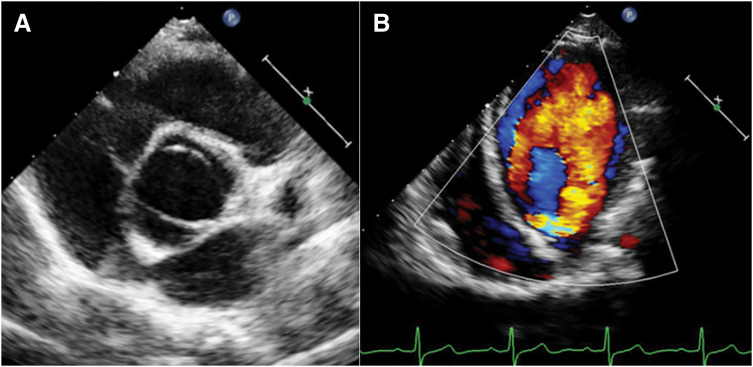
Preoperative echocardiography findings. (A) Short-axis view showing the bicuspid aortic valve. (B) Four-chamber view showing a severe aortic regurgitation jet swirling within the left ventricle.
